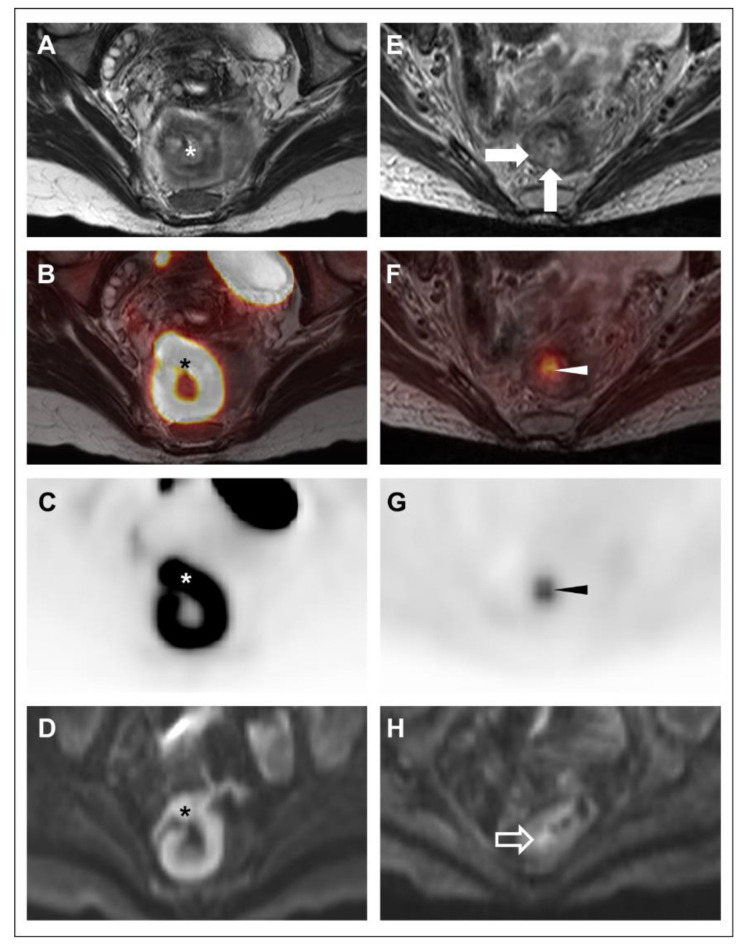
39-year-old woman with rectal adenocarcinoma (Subject #7) underwent FDG-PET/MRI at initial staging (A–D) and following TNT (E–H). Oblique axial high-resolution coronal T2-weighted images (A,E); oblique axial high-resolution coronal T2-weighted images with PET fusion (B,F), oblique axial attenuation-corrected PET images (C,G), and axial diffusion-weighted images (b = 1000 s/mm^2^) (D,H) are shown. At initial staging, a hypermetabolic, diffusion-restricting high rectal mass (*) was noted. There was no definite T3 disease, though assessment was limited by motion artifact and partial intussusception of the lesion. No suspicious lymph nodes were identified. Based on the limitations in T-stage determination, the patient underwent TNT. On restaging, both readers suspected some residual disease on MRI based on nodular T2-intermediate wall-thickening (closed arrows) and focal diffusion restriction (open arrow). Both readers assigned an mrTRG of 3. On the PET, there was a clear focus of hypermetabolism (arrowheads) in this same area, and both readers assigned a pmrTRG of 3. Although residual disease was already suspected based on the MRI alone, both readers reported a substantial increase in confidence based on the PET findings. Endoscopic biopsy confirmed residual disease, and the patient underwent resection with pathology showing ypT2 ypN0 disease.
